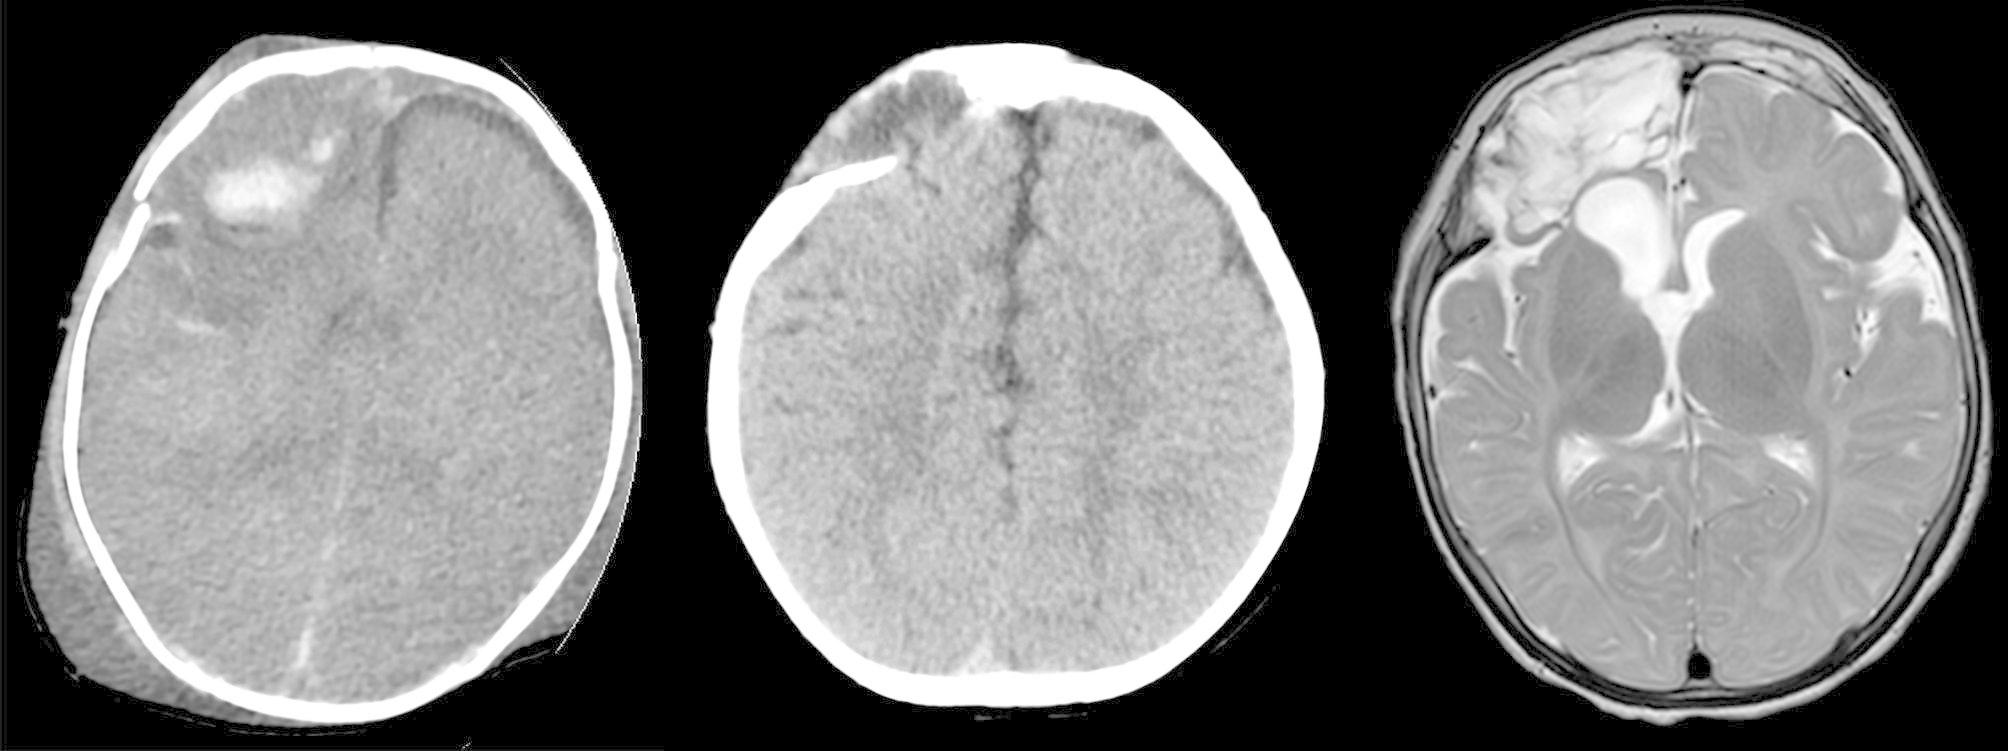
Growing skull fracture, CT and MRI scan. Left image, linear fracture immediately posttrauma, right frontal lobe. Middle and right image, 7 months later, note the herniation of the skull defect and degenerated brain tissue
